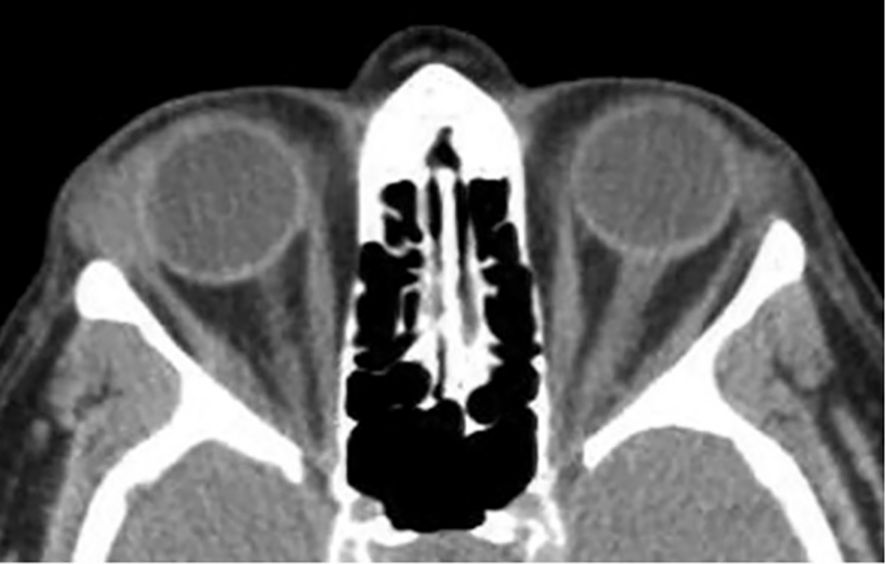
Axial non-contrast CT image of the orbits in a 71-year-old female patient showing diffuse enlargement of the right lacrimal gland with relatively homogeneous soft tissue attenuation and mild adjacent preseptal fat stranding, a hazy, poorly defined increase in fat attenuation that reflects edema or inflammation. The findings are compatible with dacryoadenitis. The diagnosis was confirmed on biopsy.
